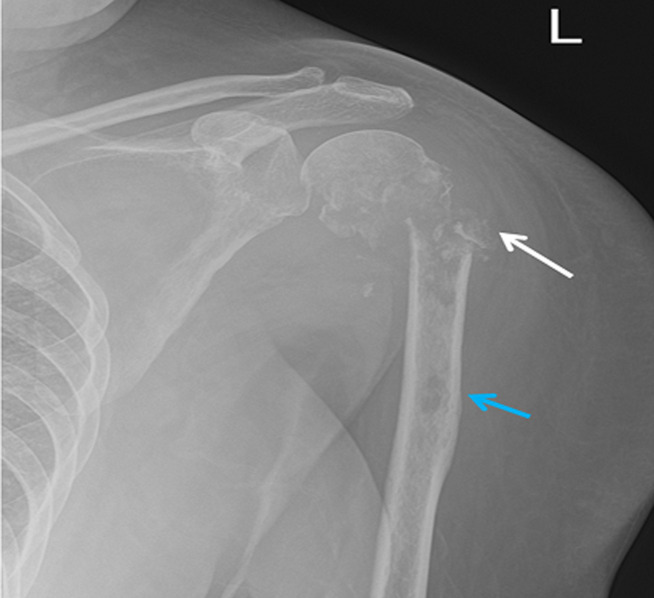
preoperative X-ray of left shoulder showing pathological comminuted fracture of proximal humerus (involving neck, greater and lesser tuberosities) with extensive lytic areas and cortical destruction of the metadiaphyseal region of the humerus (white arrow), and another intramedullary lytic area at the proximal diaphysis (blue arrow)
